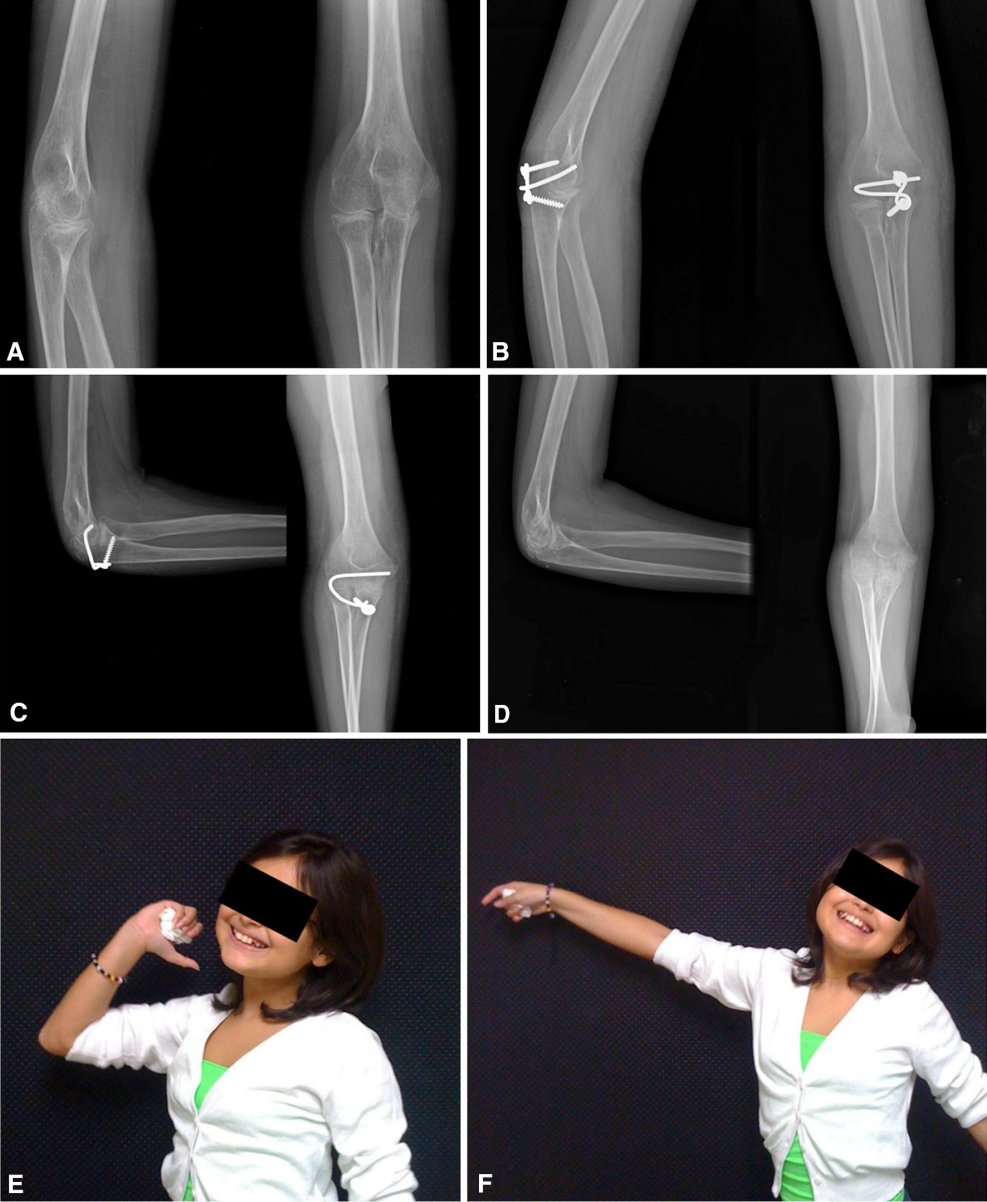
(A) This is a preoperative radiograph of a 9-year-old girl who has juvenile rheumatoid arthritis with 6 months’ duration of complete loss of elbow motion. The elbow was fixed in full extension, having failed all forms of conservative treatment. (B) After full release and takedown of an early synostosis, the elbow was stabilized with the joint stabilizer implant. (C) At 4 weeks, a painful proximal screw head and implant prominence were delaying rehabilitation; therefore, the proximal screw and proximal part of the implant were removed. (D) Radiographic images of the elbow 2 years after the final removal of the implant are shown. (E) Elbow flexion and (F) extension are shown 2 years postimplant removal.
